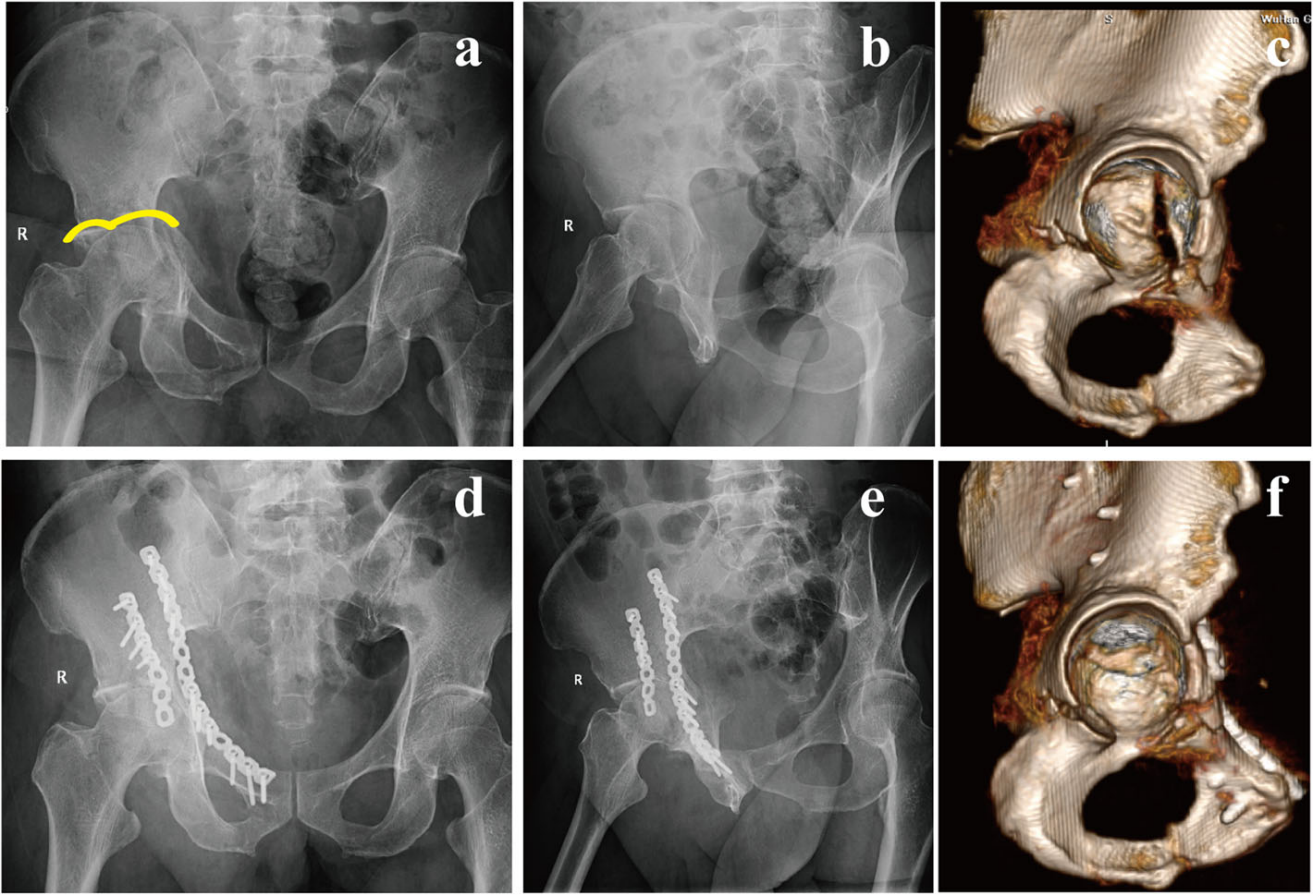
A 61-year-old man presented with acetabular fracture of the right acetabulum. Preoperative AP view (a) showed a typical feature of “Gull sign”. Judet view (b) and 3D CT reconstruction (c) showed that the quadrilateral plate was separated from the anterior column and partially attached to the posterior column, which was classified as QLP1. ORIF was performed via a single ilioinguinal approach, and the compression fracture of acetabular dome was managed with artificial bone grafting. Post-operative X-rays (d, e) and 3D view (f) showing an anatomical reduction
